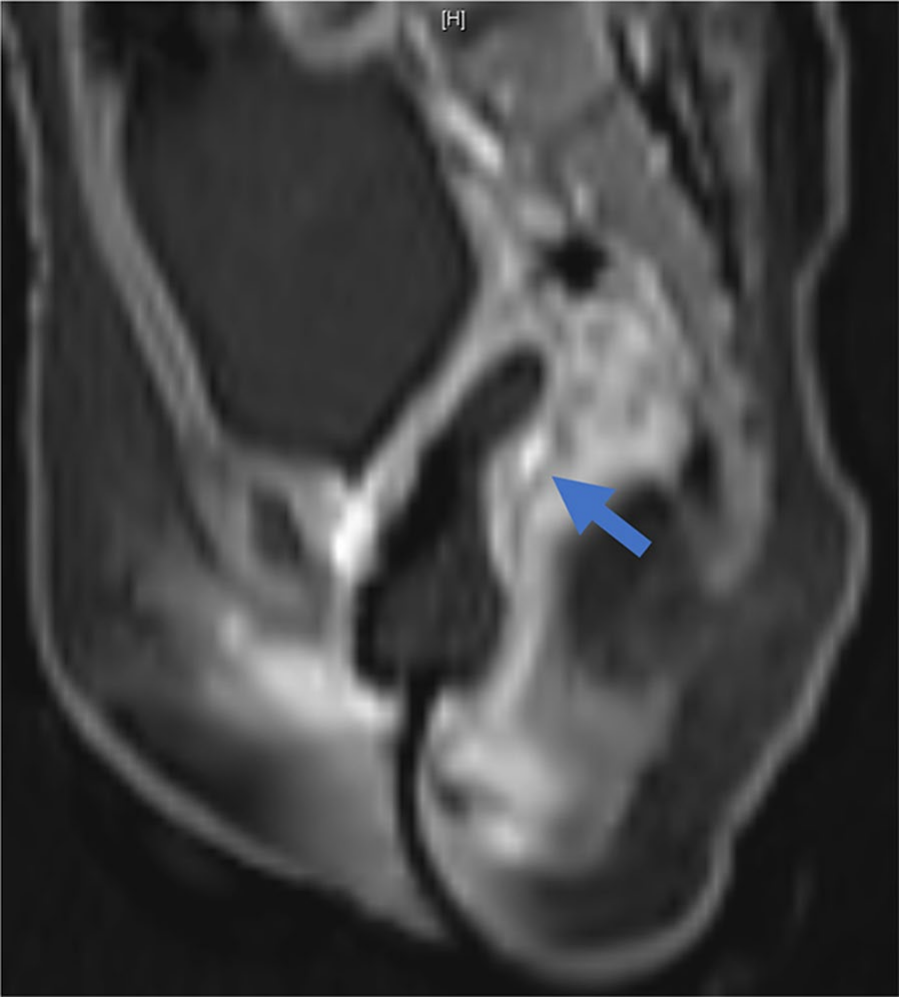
Gadolinium-enhanced magnetic resonance imaging of the tumor before total resection. Preoperative imaging reveals a residual tumor on the posterior wall of the vagina. The vagina is inflated with saline solution. Arrow: the tumor of interest
